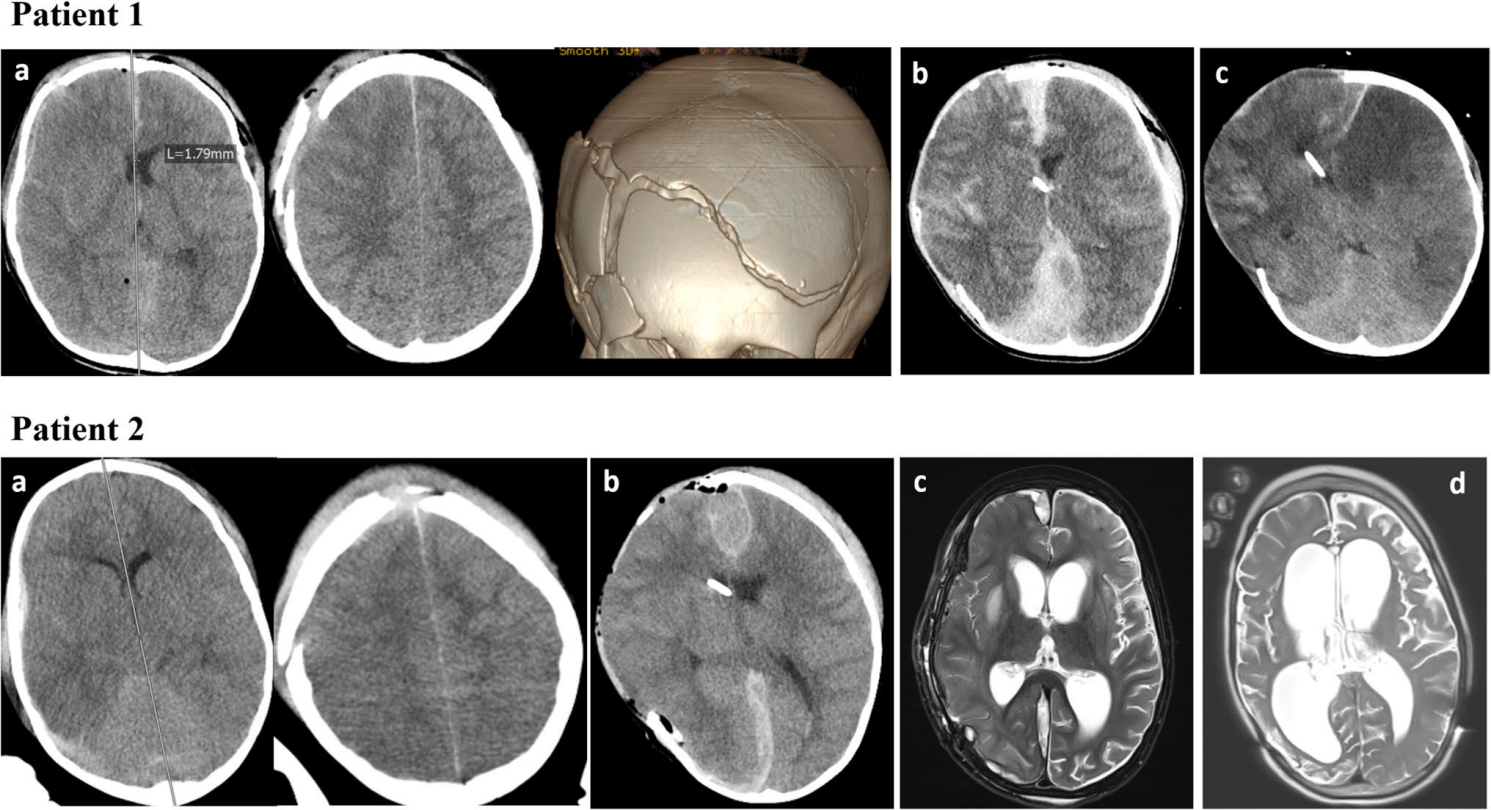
Exemplary cases of two children suffering from severe TBI and treated with decompressive craniectomy. Patient 1 was a 4-year-old boy suffering from a direct hit against the skull through the mirror of a past-driving car. The child presented with GCS 3, anisocoria, and non-intact light reaction. The initial CT scan (a) showed a complex frontal skull fracture and a diffuse TBI with a 2-mm midline shift. The child was treated with unilateral decompressive hemicraniectomy, and an EVD and an ICP probe were placed (postop scan: b). ICP was critically elevated throughout the postoperative course (ICP_max._ 40 mmHg), and the child presented with severe infarction and brain death at 14 days postop (C). Patient 2 is a 9-month-old boy who fell from a height and also presented with GCS 3, anisocoria, and non-intact light reaction. The CT scan (a) showed a right frontal skull fracture and a diffuse TBI without midline shift. The child was also treated with unilateral decompressive hemicraniectomy, and an EVD and an ICP-probe were placed. ICP_max_. was 21 mmHg (postop scan: b). Cranioplasty with reimplantation of the formerly removed bone flap was performed 21 days postop (c) without complications. The child showed brain hypotrophy in the follow-up scans (d, 2-year follow-up) and lives with respective disabilities (GOS 4)
